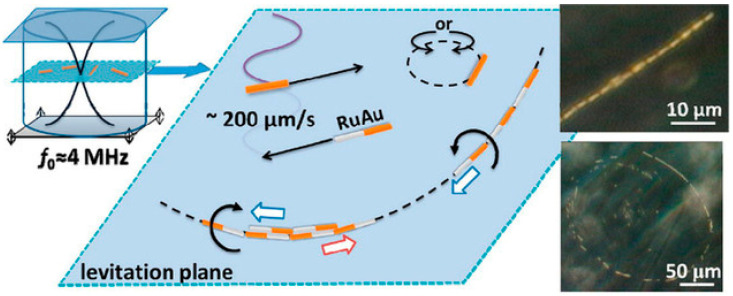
Ultrasonic standing wave powered Au/Ru rods. The figure has been reproduced with permission from the American Chemical Society [163].
